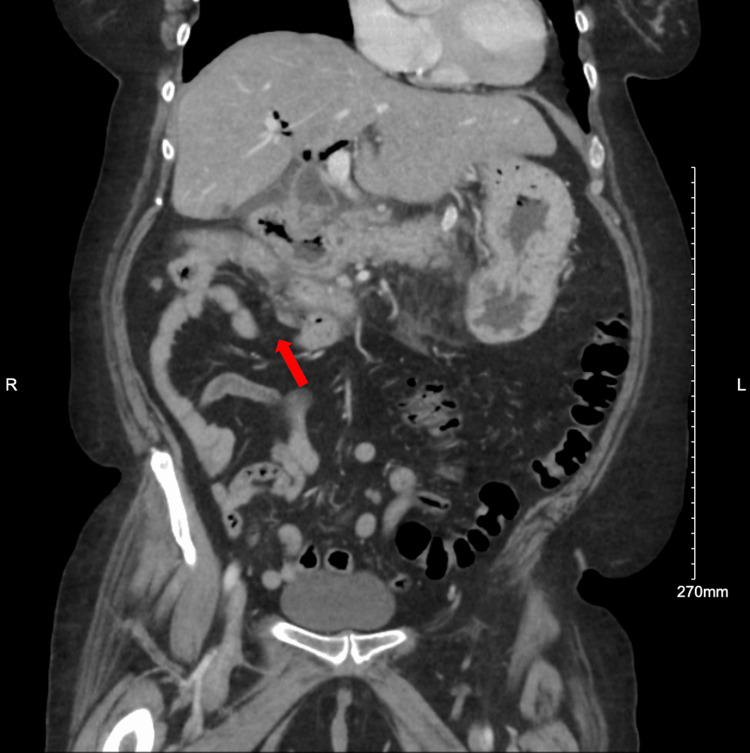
Coronal computed tomography (CT) abdomen/pelvis with contrast. Arrow points to a malrotated duodenum in the right upper quadrant of the abdomen.
